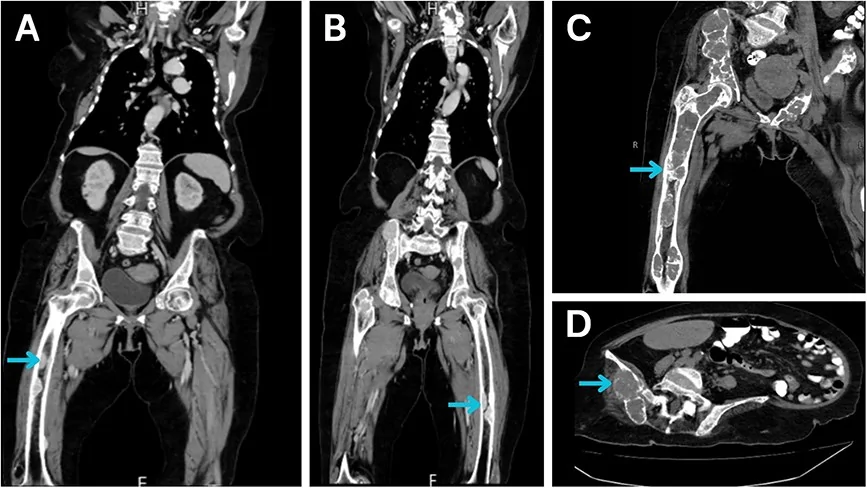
(A–C) Coronal views of post-contrast CT scan shows rounded cortically based lesions (arrows) in both femurs. (D) Axial view of CT scan without contrast of the pelvis shows expansile lesions (arrow) in the right iliac bone.
